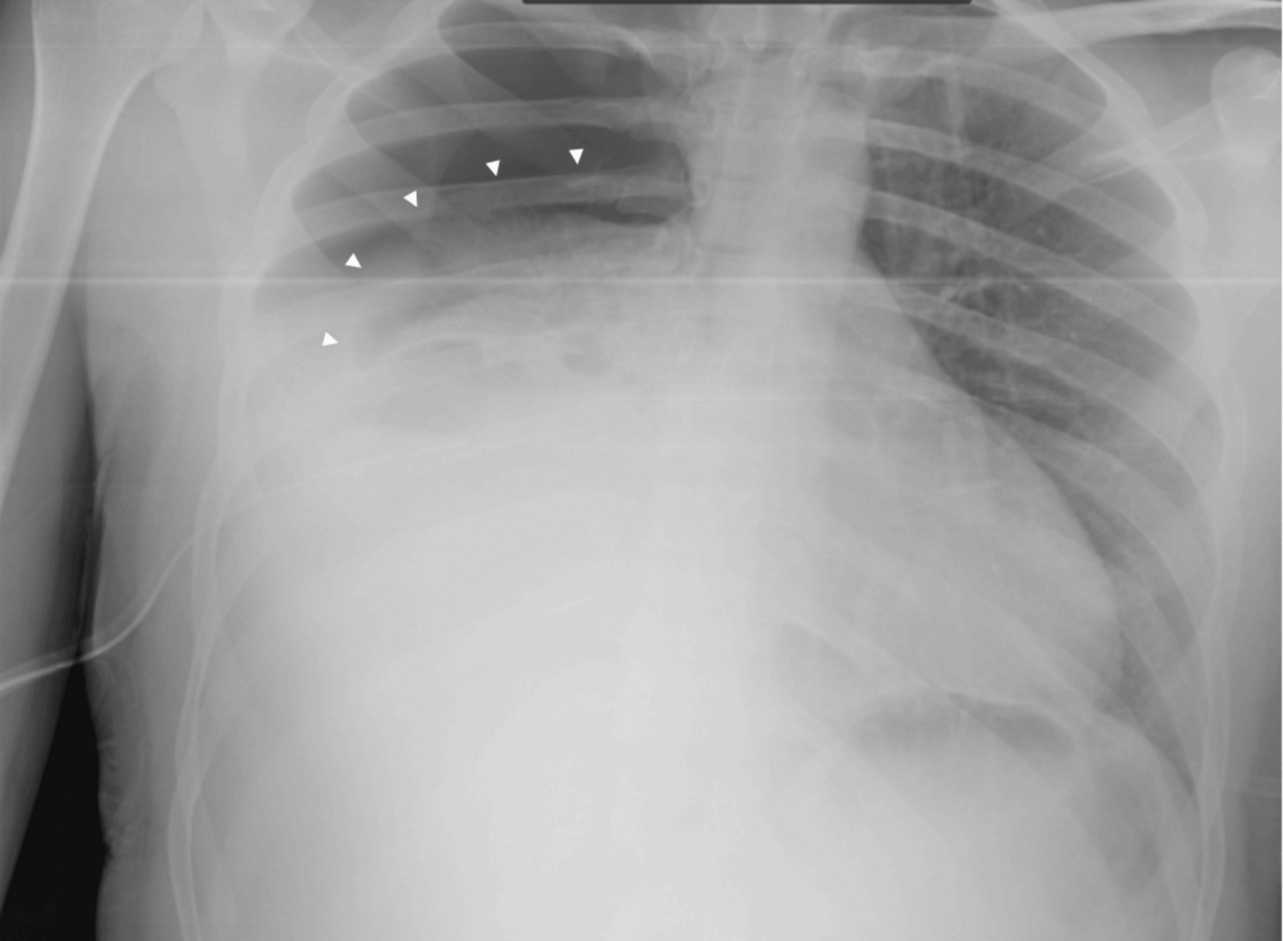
Post-drainage chest radiograph Radiograph after placement of a right-sided chest tube showing worsening of the pneumothorax and pleural effusion (white arrowheads outline the margin of the collapsed right lung).
